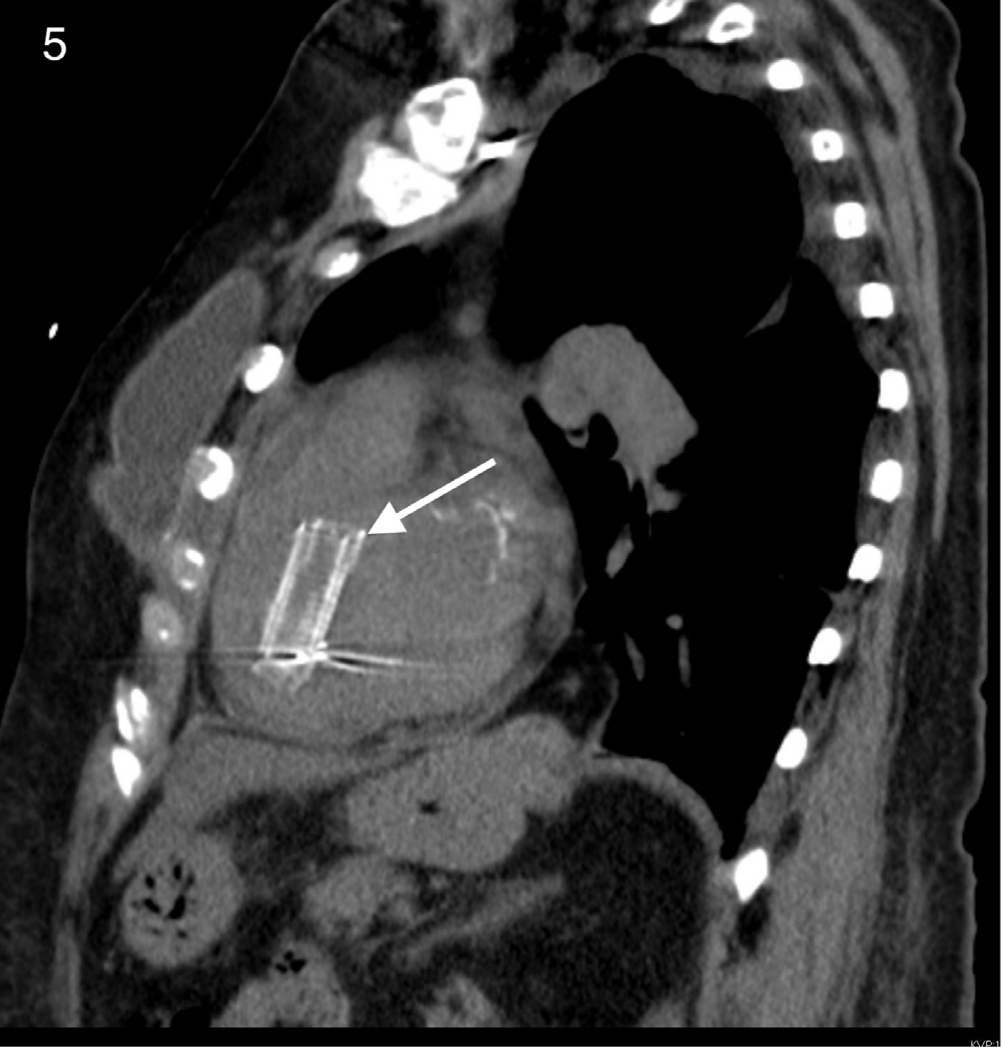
Sagittal view CT demonstrating a migrated stent in the right ventricular outflow tract (white arrow).
